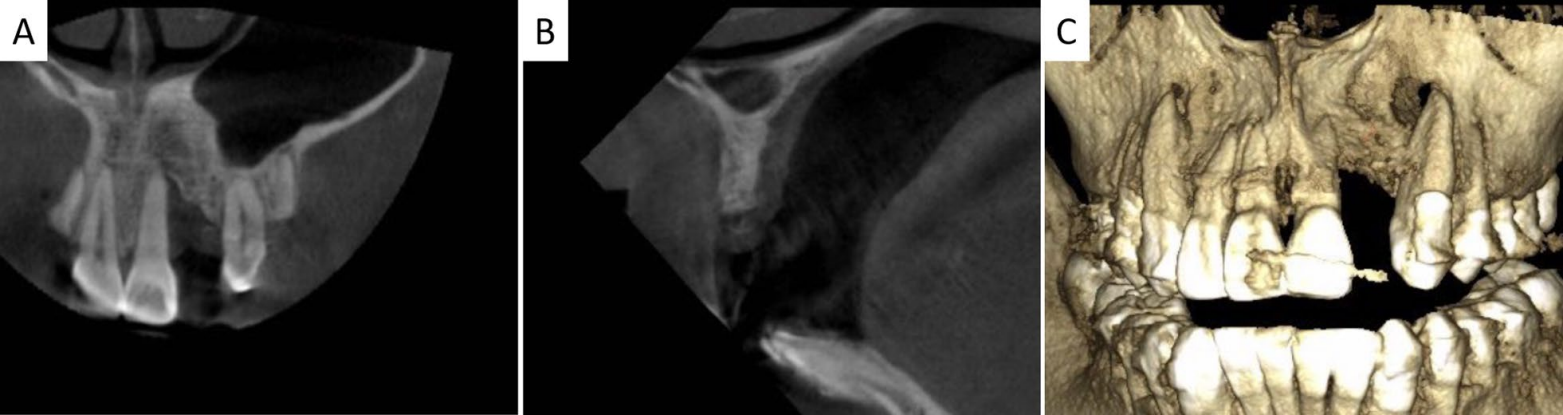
CBCT examination before surgery. A–C showed coronal-, sagittal plane and 3D reconstruction views
